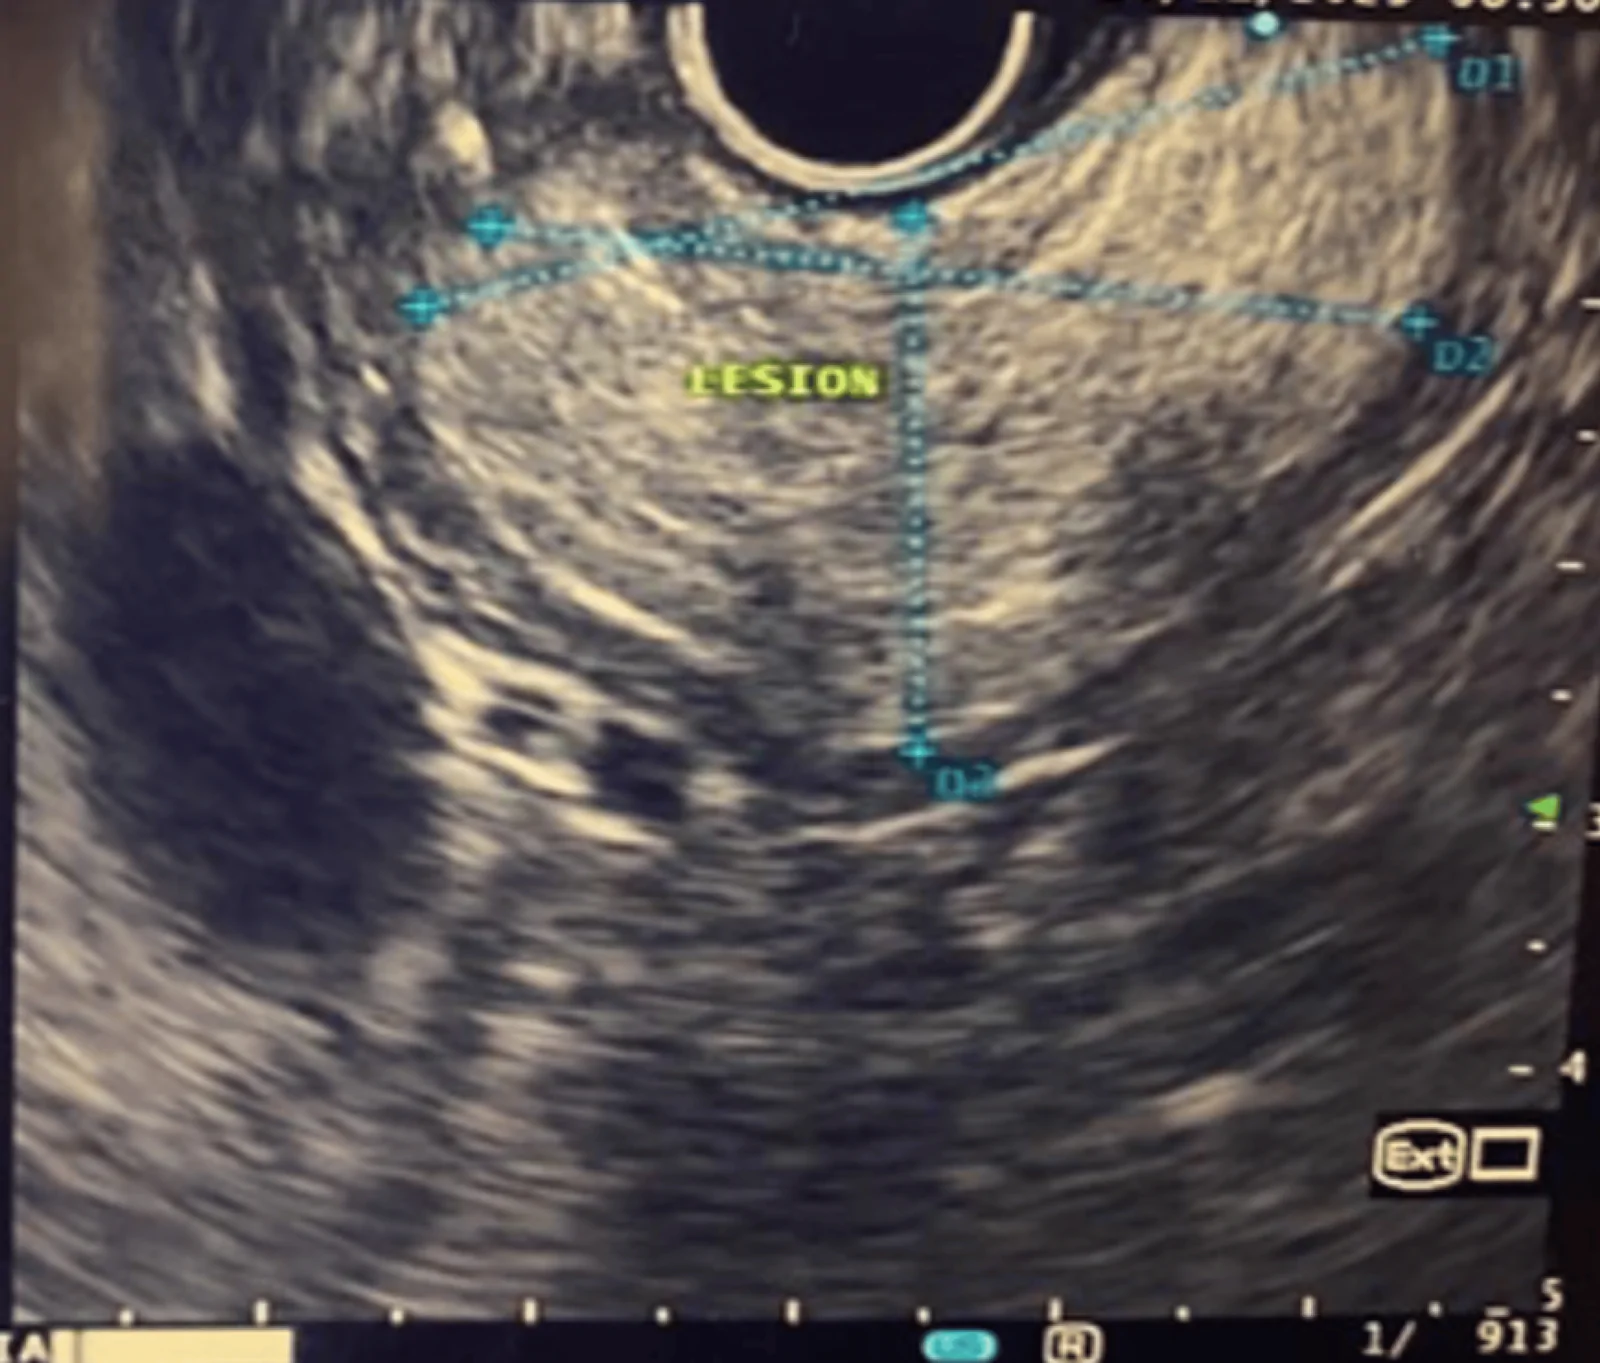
EUS showing a homogeneous, hyperechoic, well-defined submucosal mass measuring 5 × 3 × 2 cm, without regional adenopathy EUS: endoscopic ultrasound
